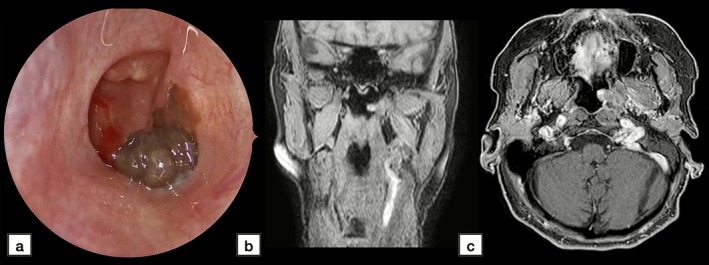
Endoscopic appearance (a) and magnetic resonance imaging (MRI) of the lesion (T1‐weighted sequence with contrast) in coronal (b) and axial (c) planes.
